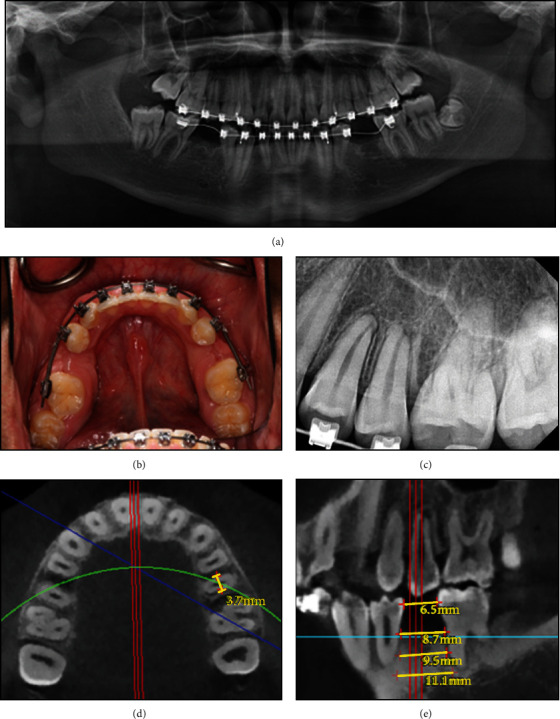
Pre-operative records of auto-transplantation. (a) Panoramic radiograph showing congenitally missing teeth #20 and #29. (b) Pre-operative periapical radiograph of tooth #13. (c) Clinical photo of mandibular arch showing congenitally missing teeth #20 and #29. (d) Pre-operative CBCT of the apical third of the root of tooth #13 (donor tooth). (e) Pre-operative CBCT of recipient site for transplanting tooth #20.
